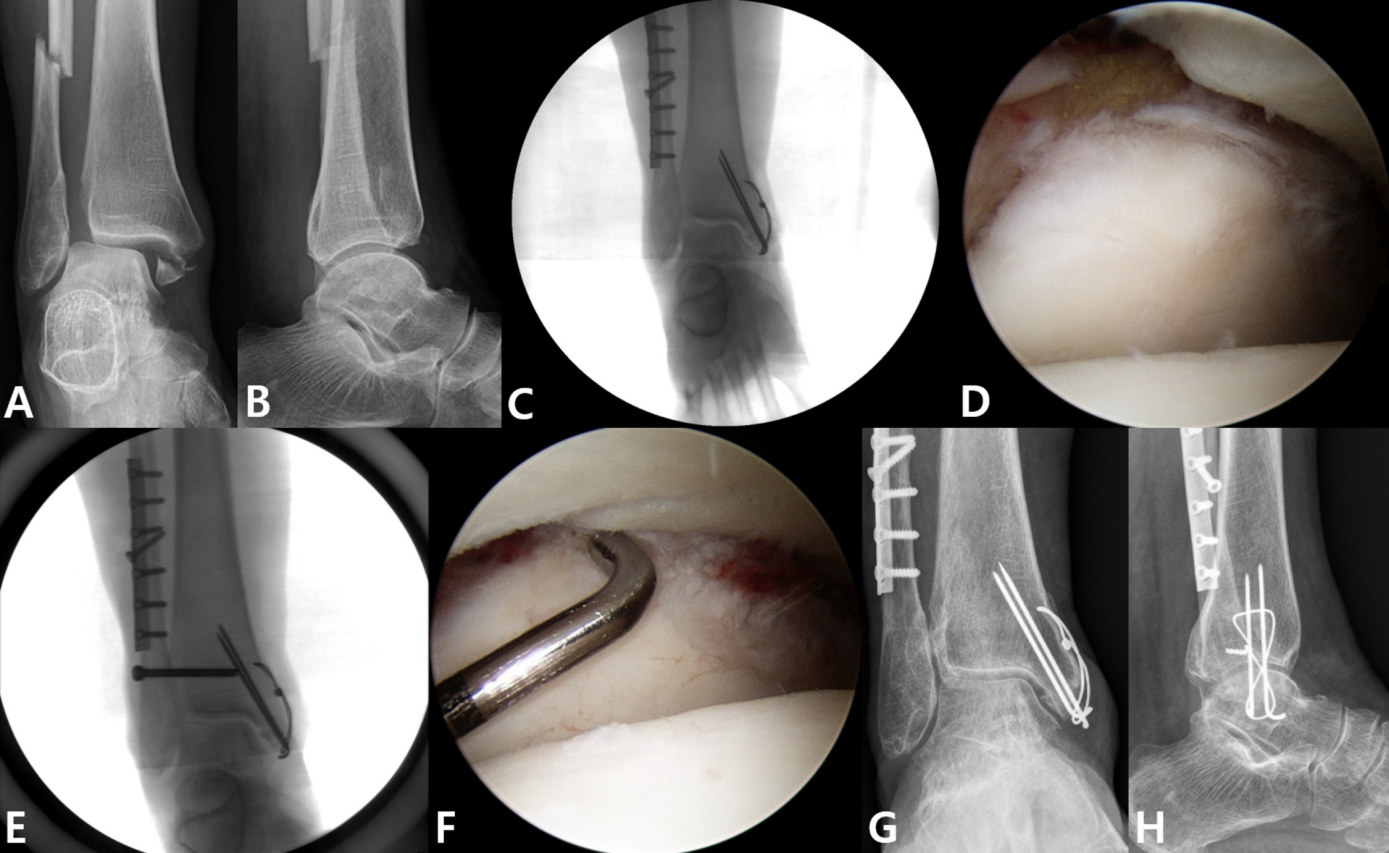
Anteroposterior (A) and lateral (B) radiographs of a pronation external rotation ankle fracture. After confirming anatomical reduction and internal fixation on the intraoperative fluoroscopic image (C), syndesmotic instability was observed through an external rotation stress test performed during arthroscopy (D). After syndesmotic transfixation (E), the stability of syndesmosis was restored (F). The syndesmotic screw was removed after three months, and anteroposterior (G) and lateral (H) radiographs taken 24 months after surgery showing successful bone union without syndesmotic widening.
